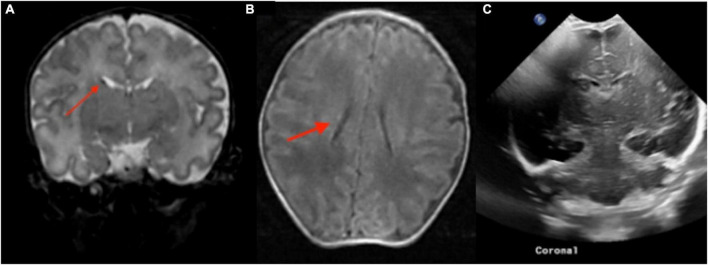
The point of care 1-Tesla MRI in a 6-day-old infant with emergency cesarean section delivery due to decreased fetal movement and variable decelerations showed a possible punctate focus of hemorrhage versus vessel in the right caudothalamic groove with T2 hypointensity (A) and T1 hyperintensity (B) but no evidence of ischemic injury. This was not seen on transcranial ultrasound on the first day after birth (C).
